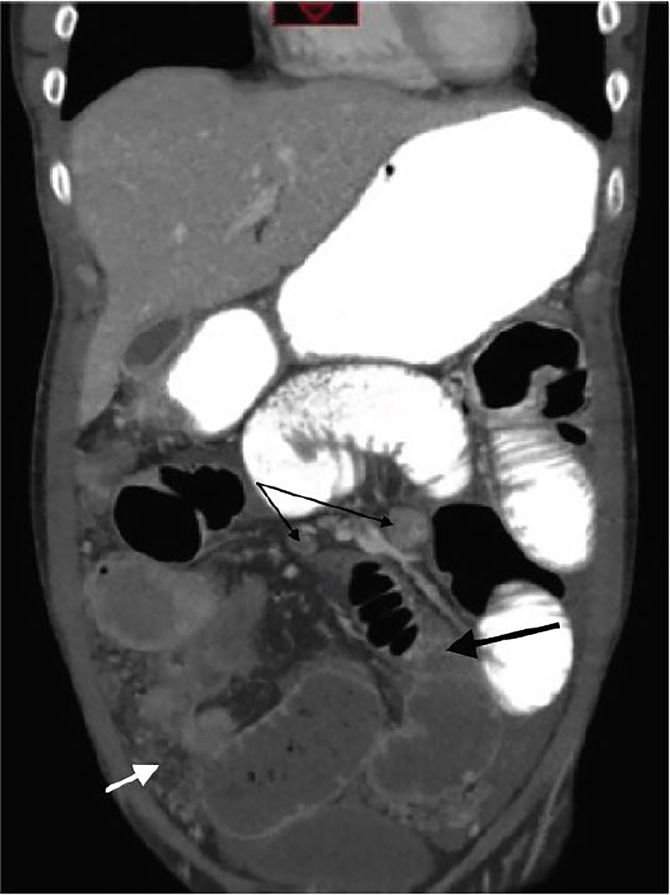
Coronal computed tomography with oral contrast showing oral contrast not completing its path through the bowel (thick black arrow), indicating presence of high-grade obstruction. Also visualized are large mesenteric lymph nodes (thin black arrows), peritoneal studding, and omental caking (white arrow).
